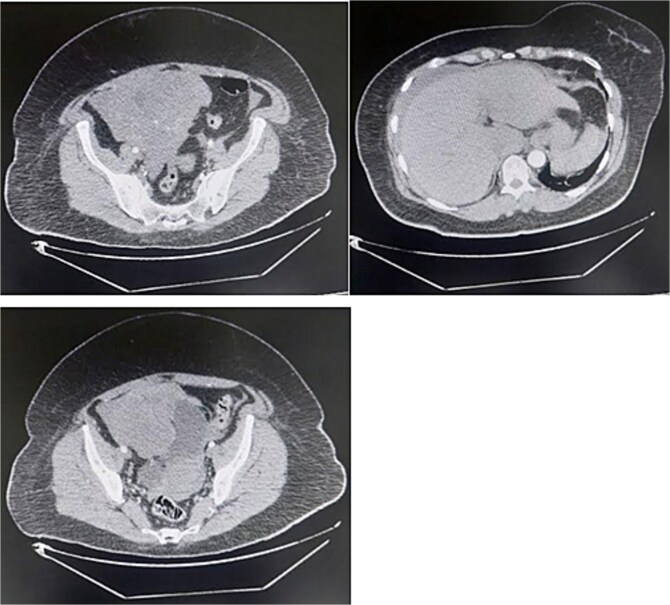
CT Scan showing the tumour mass with massive peritoneal effusion in our patient.
